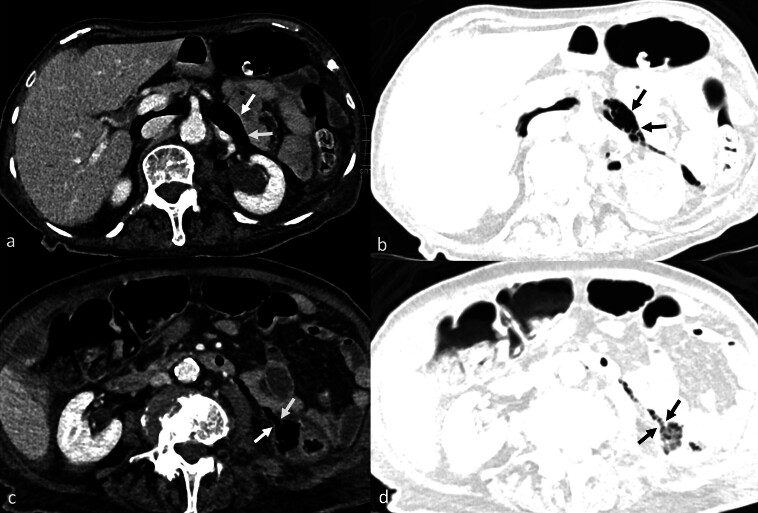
Axial CT images of the abdomen.
